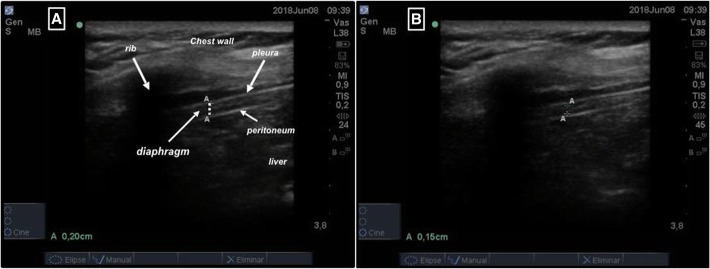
Representative ultrasound image at the zone of apposition in B-mode view of the diaphragm during inspiration (a) and expiration (b). The diaphragm is identified as a 3-layer comprising two hyperechoic lines representing the pleural and peritoneal membranes and a middle hypoechoic layer representing the diaphragmatic muscle itself (*with permission of the Intensive Care Unit from the Hospital Universitario de Getafe)*
